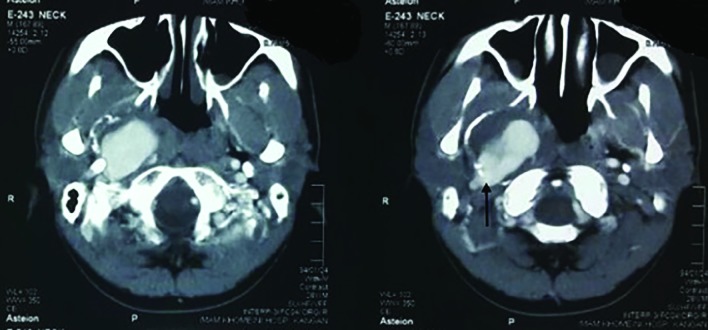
Axial computed tomography (CT) angiogram of the patient revealed a huge aneurysm at the distal segment of the internal carotid artery. The aneurysm is injured as demonstrated by extravasation of the contrast material (Arrow)
